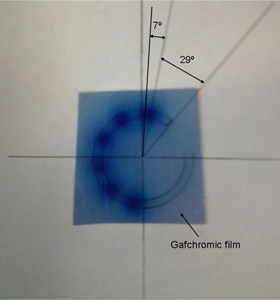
Radiograph of the Ir‐192 source in dwell positions 1 to 6, respectively. A 30° ring applicator is in use and is placed in the apparatus with alignment, according to the drawn template shown in Fig. 1, with a piece of GAFCHROMIC film under it.
